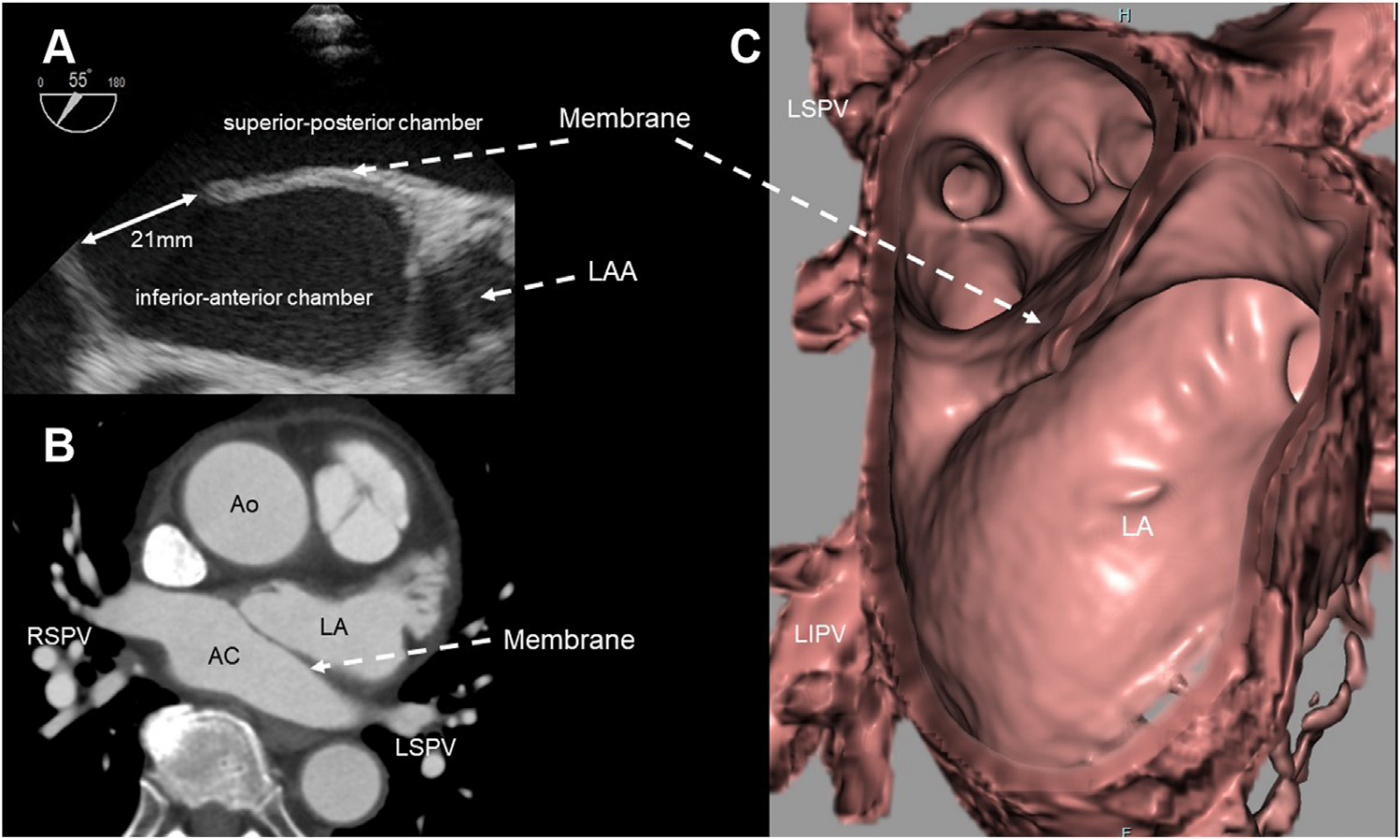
Images obtained via transesophageal echocardiography (A) and multidetector computed tomography (B, C). The fibromuscular membrane divided the left atrium into 2 chambers. The pulmonary veins opened into the superior and posterior accessory chambers, connected via the fibromuscular membrane to the inferior and anterior chamber and the main left atrial chamber. AC = accessory chamber; Ao = aorta; LA = left atrium; LAA = left atrial appendage; LIPV = left inferior pulmonary vein; LSPV = left superior pulmonary vein; RSPV = right superior pulmonary vein.
